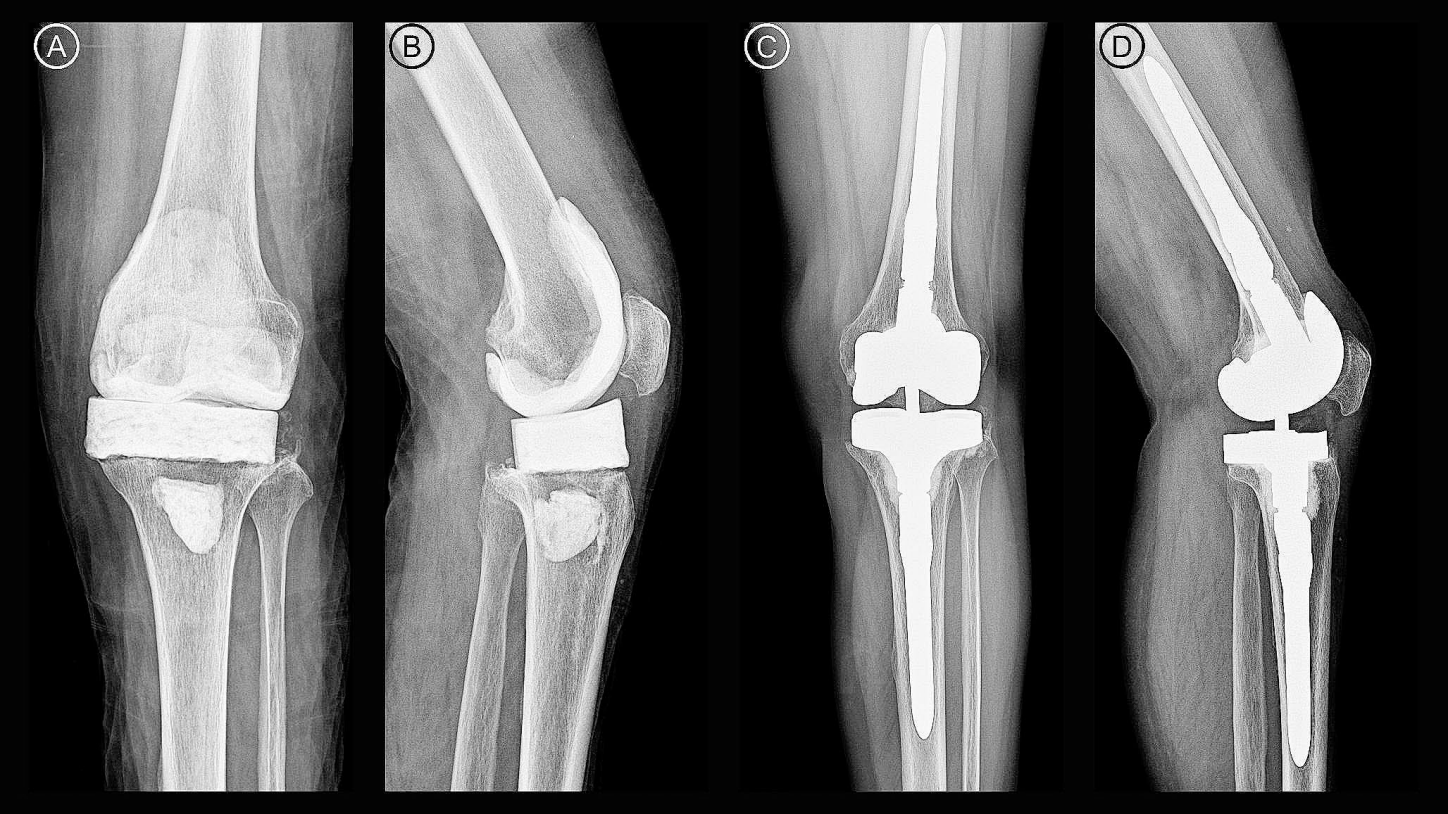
(A) Anteroposterior and (B) lateral radiographs obtained at first-stage revision arthroplasty using a spiked tibial cement spacer (C) anteroposterior and (D) lateral radiographs obtained at 21 months after second-stage revision arthroplasty using a revision system
